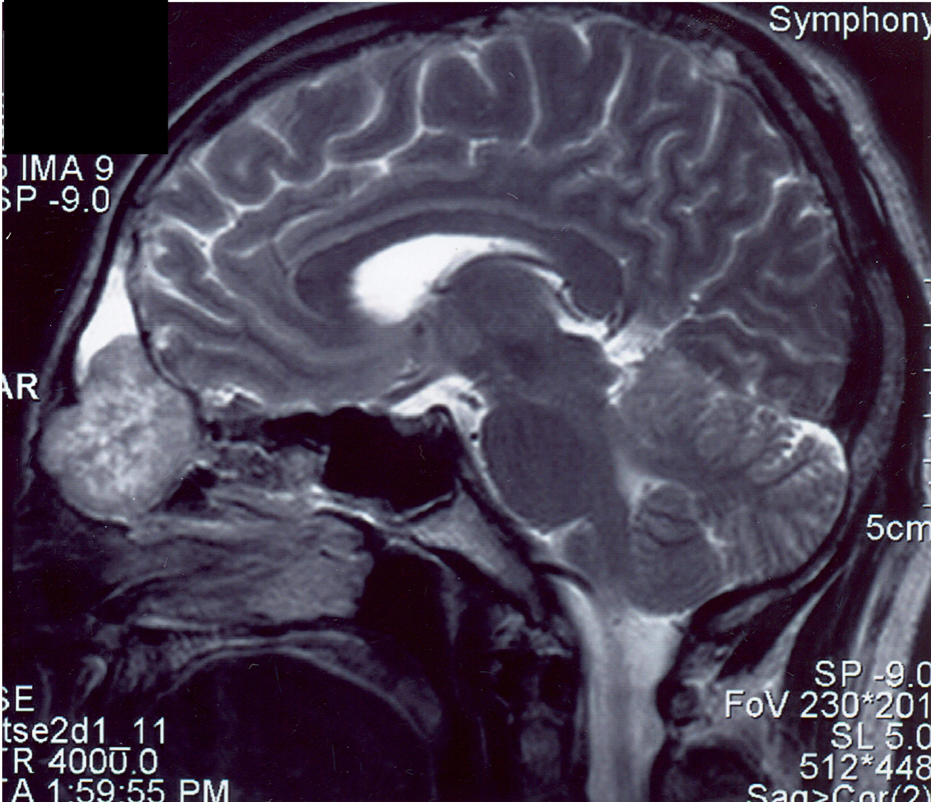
T2W MRI sagittal scan. Well bordered tumorous masses of the nasal cavity, heterogeneous, and more hyperintensive structures, destruction of the surrounding skeleton, prominence of the masses into the bases of frontal sinuses, the rest filled with liquid. No intra-cranial growth - lamina interna of the frontal sinus preserved - narrow hem with no signal.
